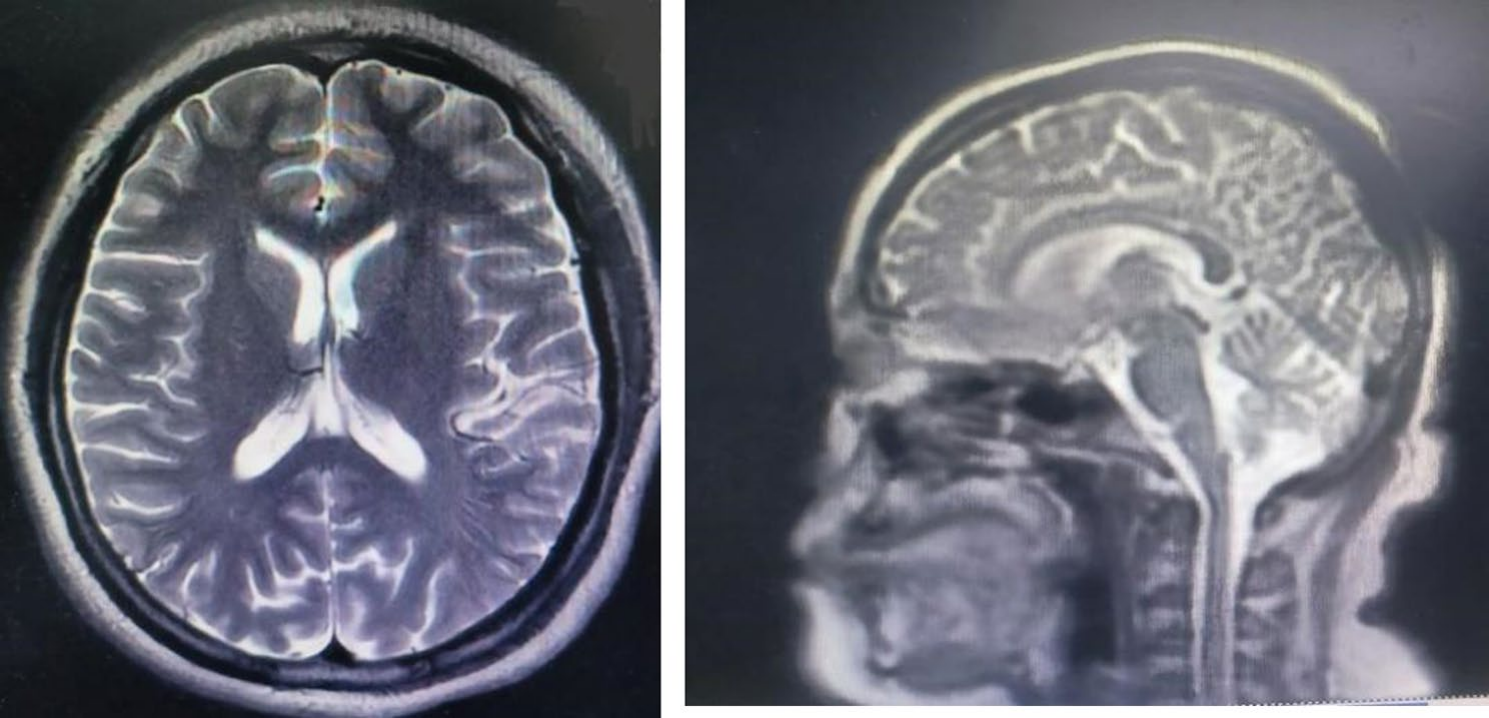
Preoperative cranial MRI
